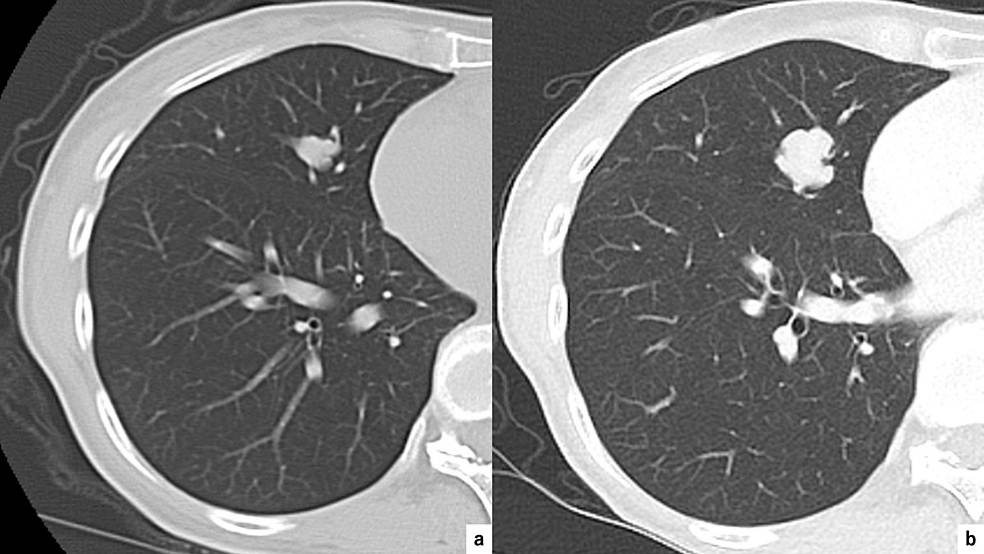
Chest CT findings (a) A chest CT scan taken by a previous doctor 10 years ago shows a nodule in the right middle lobe. (b) The nodule enlarged gradually up to 3.1cm of maximum diameter over 10 years.
